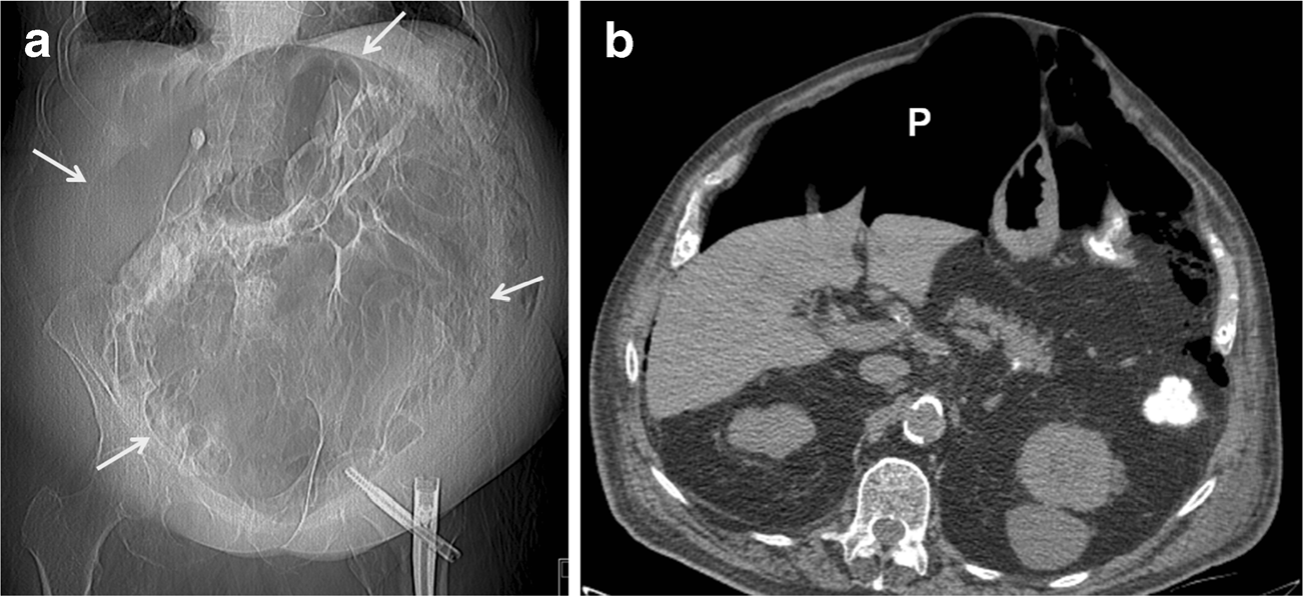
A 92-year-old man with massive pneumoperitoneum due to left colon perforation. CT surview of the abdomen (a) demonstrating the football sign (arrows) that may mimic giant colonic diverticulum. Axial unenhanced CT image through the upper abdomen (b) shows the massive pneumoperitoneum (P)
